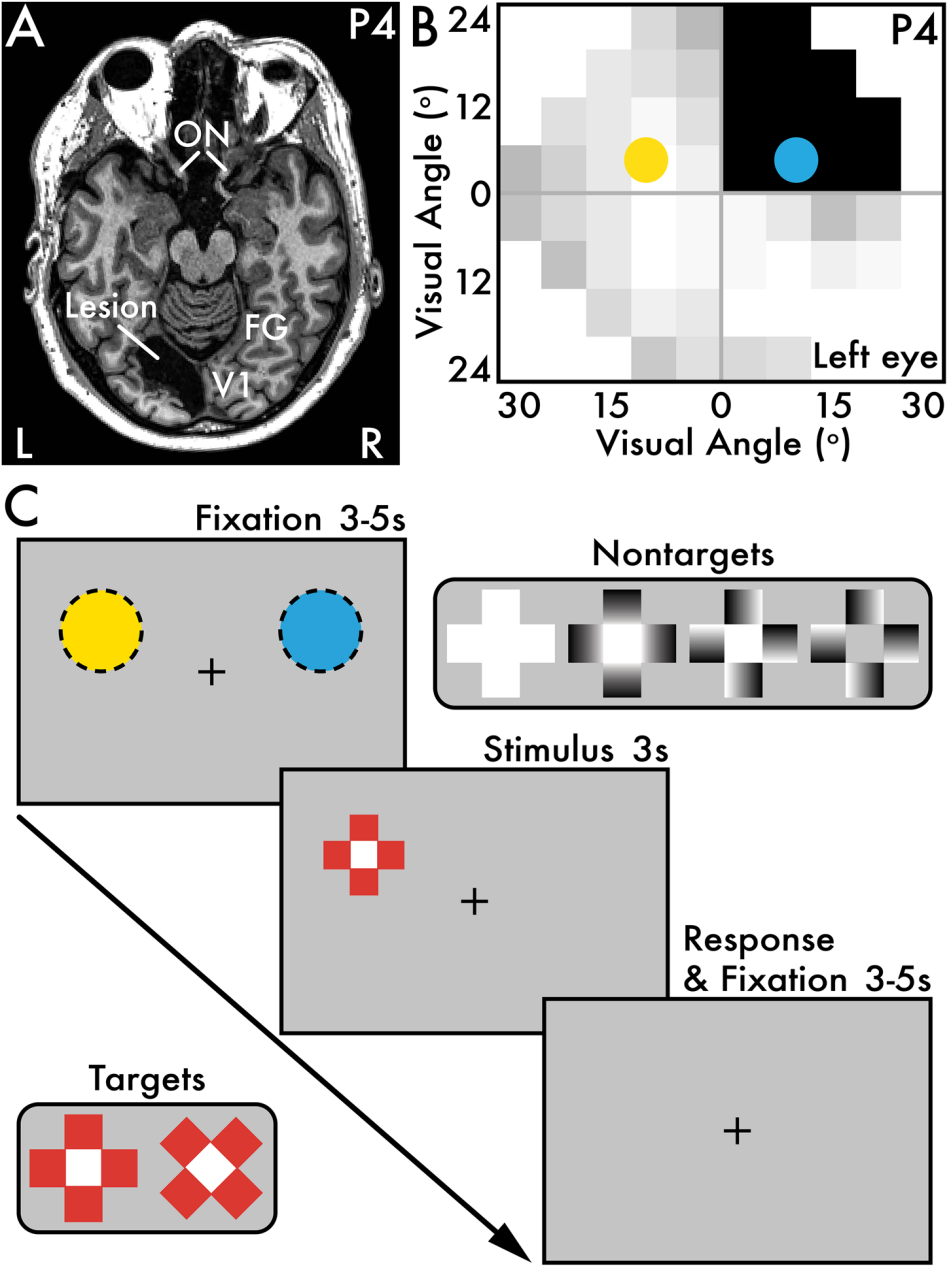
Patient participant P4 stroke location and Humphrey visual field test results, and the visual perception task.* A Patient participant P4 (Table 1) axial T1-weighted whole brain anatomical MRI scan revealing a lesion in the left primary visual cortex (V1) affecting regions ventral to the calcarine sulcus. The right (R) cortical hemisphere is intact. Major primary visual pathway anatomical landmarks are labeled: optic nerve (ON), V1, and fusiform gyrus (FG). B P4’s Humphrey visual field (HVF) test results from the left eye in degrees (^o^) of visual angle indicates a right homonymous superior quadrantanopia. Sighted areas are indicated by white and light gray squares, while blind areas are indicated by dark gray and black squares. The colored circles (sighted field: yellow; blind field: blue) indicate the on-screen stimulus presentation locations for the visual perception task. C Each visual perception task trial involved three main phases: (1) a pre-stimulus fixation period (3–5 seconds [s]), (2) a stimulus presentation period (3 s), and (3) a post-stimulus response and fixation period (3–5 s). Throughout all phases within a trial, participants were instructed to maintain fixation on the central plus sign image. There were two categories of stimuli: (1) *target* and (2) *nontarget*. The target stimuli were red with a white center and appeared in equal proportion between two orientations: (1) plus sign (+) and (2) x-oriented. Participants were instructed to make an immediate keypress upon perceiving the target stimulus regardless of its perceived vividness (i.e., clearly versus barely seen or sensed) and indicate which orientation the target appeared. There were four nontarget stimuli that appeared in equal proportion: (1) white, (2) glare, (3) nonglare, and (4) isoluminant stimuli. Participants were instructed that no keypress was required when the nontarget stimuli appeared. The yellow (sighted) and blue (blind) dotted circles approximate the stimulus presentation locations for P4, corresponding with the circles overlaid on the HVF test results in B. The stimulus presentation locations were adjusted for each patient participant according to their visual field impairment (Table 1; Supplementary Fig. 1). Control participants were shown stimuli in the exact same on-screen locations as their paired patient participants (Table 2). Stimuli appeared in equal proportion between the two stimulus presentation locations.
